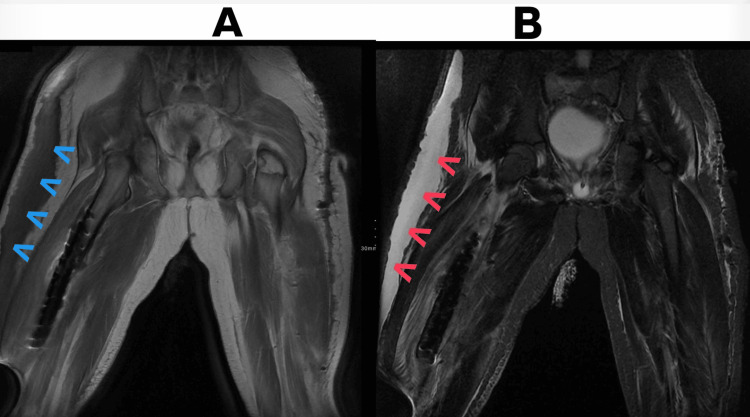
Coronal MRI of the pelvis and thighs demonstrating a Morel-Lavallée lesion. (A) Post-contrast T1-weighted image showing a fluid collection along the lateral right thigh (blue arrows). (B) Proton density fat-saturated image demonstrating corresponding high signal intensity (red arrows).
